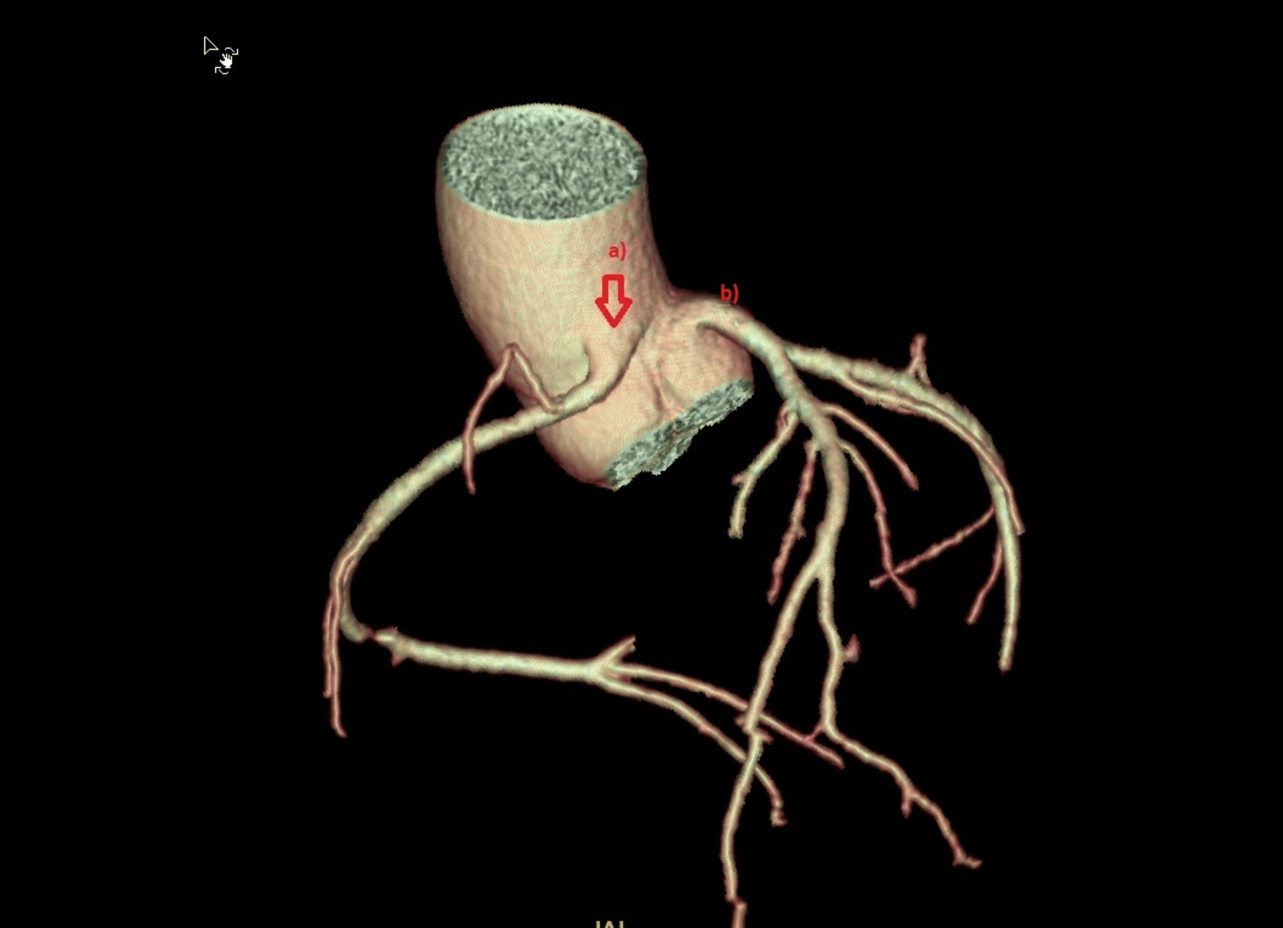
Multiplanar and 3D reconstructions of CCTA. (a) Cross-section showing the anomalous right coronary artery (arrow) arising from the aortic wall between the right and left coronary sinuses. CCTA, coronary computed tomography angiography; a, anomalous right coronary artery; b, left coronary artery origin from the left sinus
